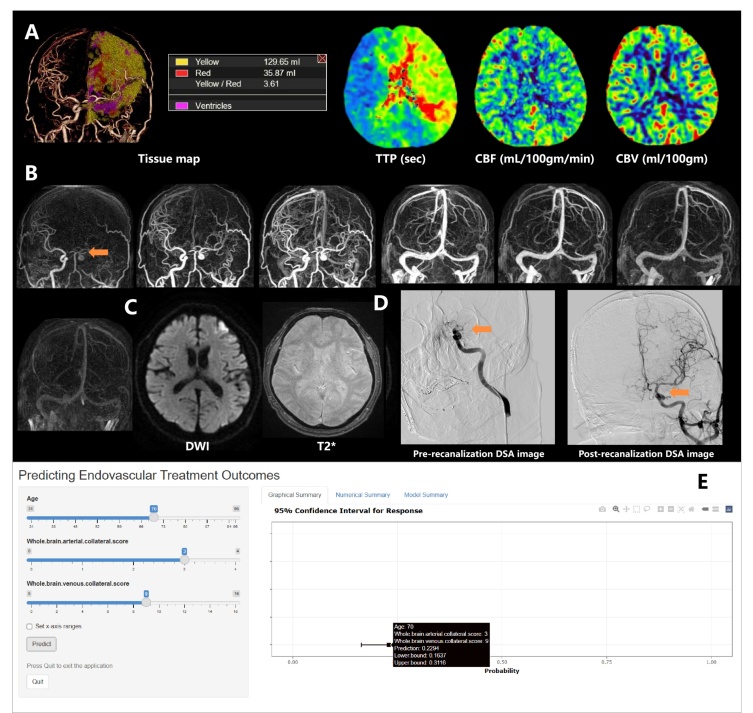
Case of effective recanalization and probability estimation. A 70-year-old patient presented with dizziness lasting 3 hours. Medical history included hypertension and liver disease. Upon admission, the NIHSS score was 1. (A-B) CTA revealed left internal carotid artery occlusion, and CTP showed prolonged TTP in the left MCA territory, with mildly reduced CBF and no significant change in CBV. The whole-brain collateral scores were 3 for arterial and 9 for venous. (C-D) After EVT, the mTICI score was 3. At 90-day follow-up, the mRS score was 0, indicating a favorable outcome and effective recanalization. (E) The dynamic prediction system assessed the risk of ineffective recanalization for this patient. The clinical parameters entered were: age 70, whole-brain arterial collateral score of 3, and venous collateral score of 9. The system predicted a 22.94% probability of ineffective recanalization, consistent with the favorable outcome.
